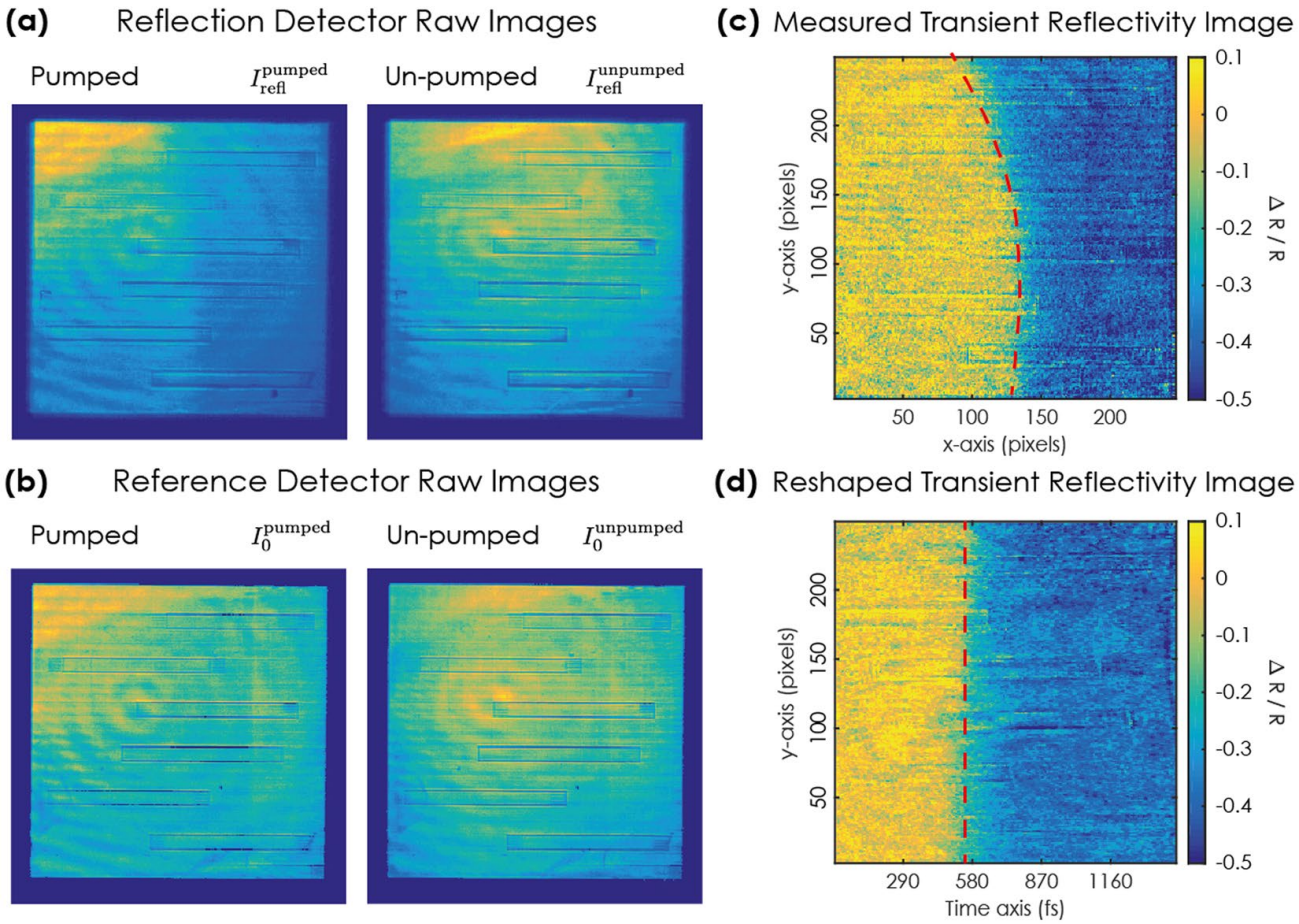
(a,b) Raw images from the reflection and reference detectors respectively. Both the images for the pumped and the un-pumped event are acquired using a single x-ray pulse. (c) Transient reflectivity image (as defined in the text) calculated from the images shown in (a,b). (d) Reshaped transient reflectivity image after calibration of the time window.
